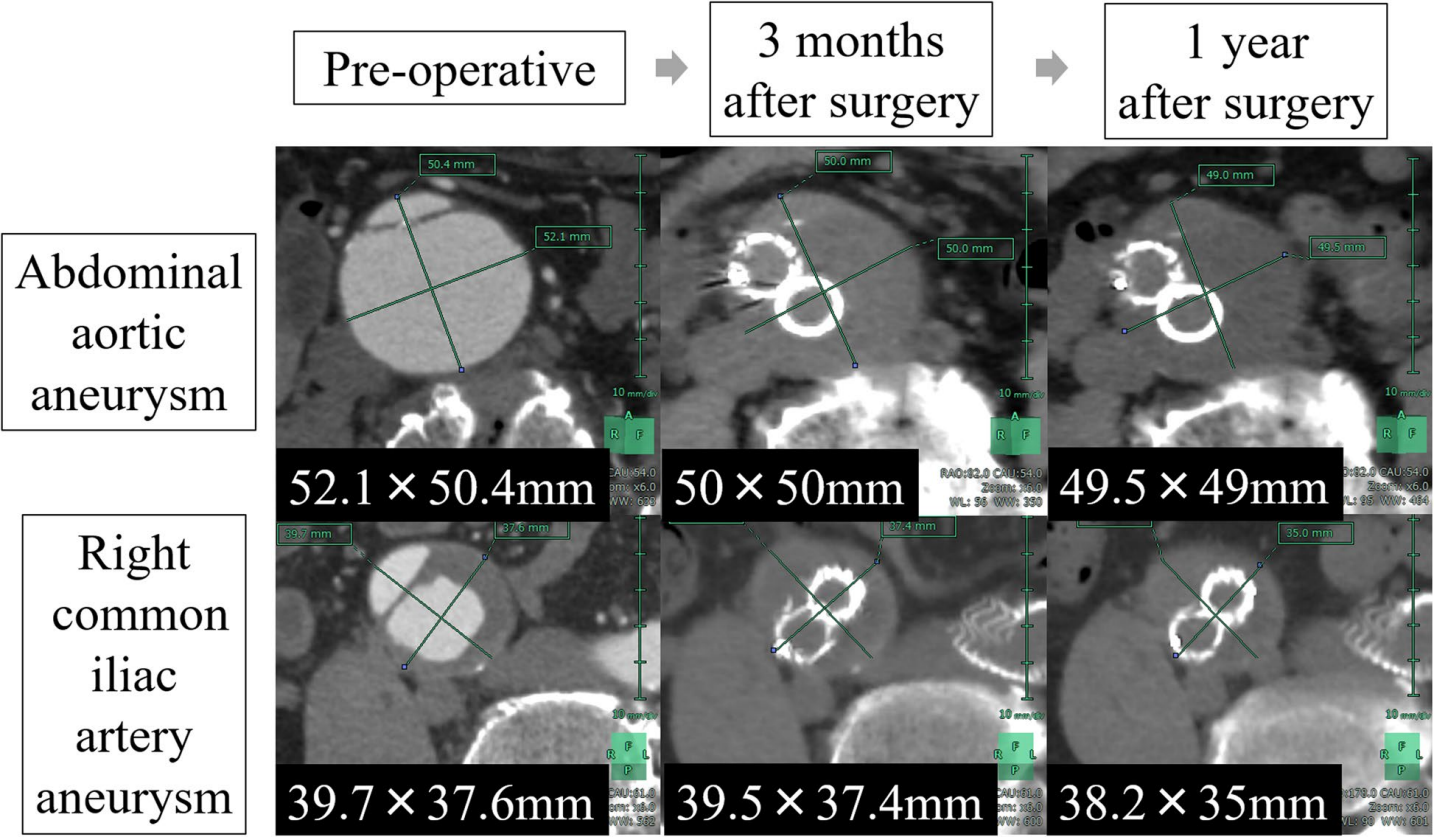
Pre- and postoperative CT images. Changes in diameter of abdominal aortic and right common iliac artery aneurysms. CT showing aneurysm shrinkage. CT, computed tomography
